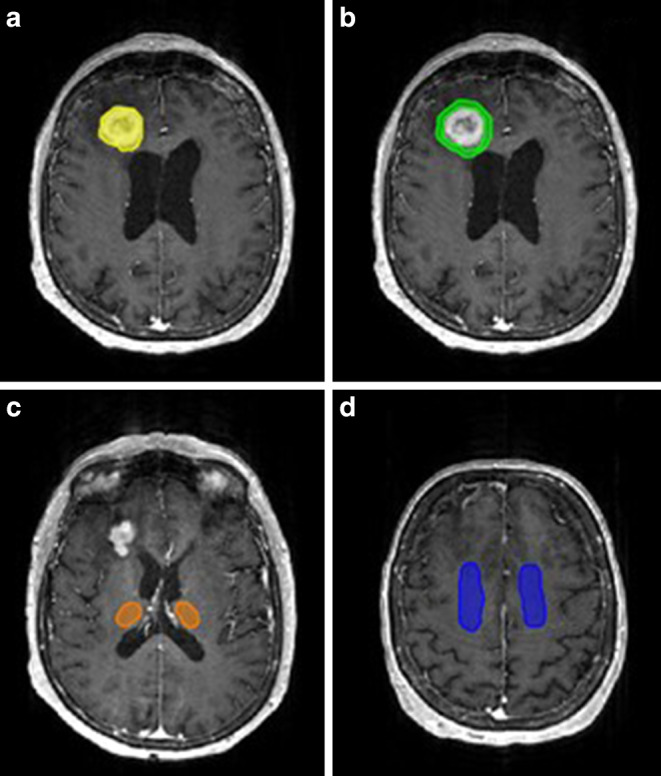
Manual selection of the regions of interest (ROIs) on postcontrast T1-weighted images. a tumor (*yellow*), b peritumoral area (*green*), c healthy gray matter from both thalami (*orange*), and d healthy white matter from the centrum semiovale (*blue*)
